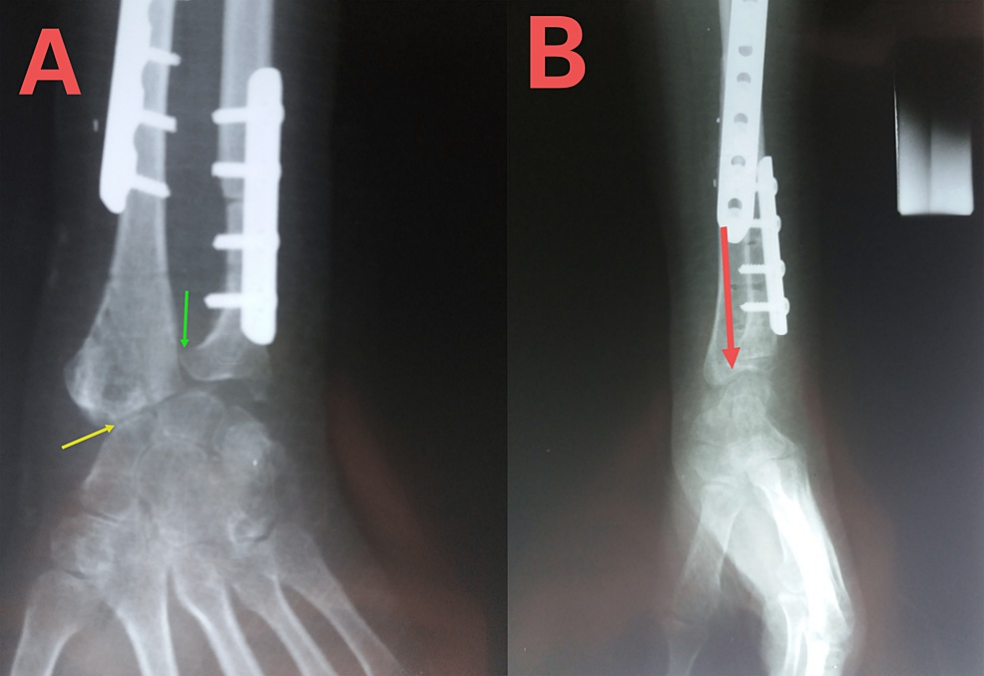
X-rays three months after the surgery. (A) Anteroposterior view, normal radio-ulnar variance (green arrow), good articulation between the graft and the proximal row of the wrist bone (yellow arrow); (B) Lateral view, good lateral alignment of the graft to wrist bones (red arrow).
